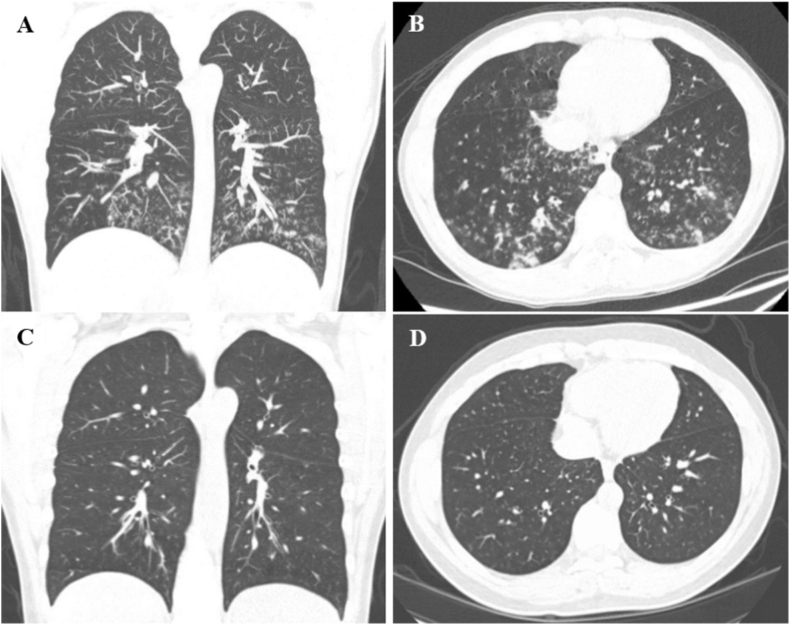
Chest CTs for case 1. A and B) Initial chest CT demonstrated innumerable patchy irregular pulmonary nodules throughout the mid and lower lungs bilaterally. C and D) Repeat chest CT 3 months after tumor debulking demonstrated resolution of the previously noted bilateral pulmonary nodules.
